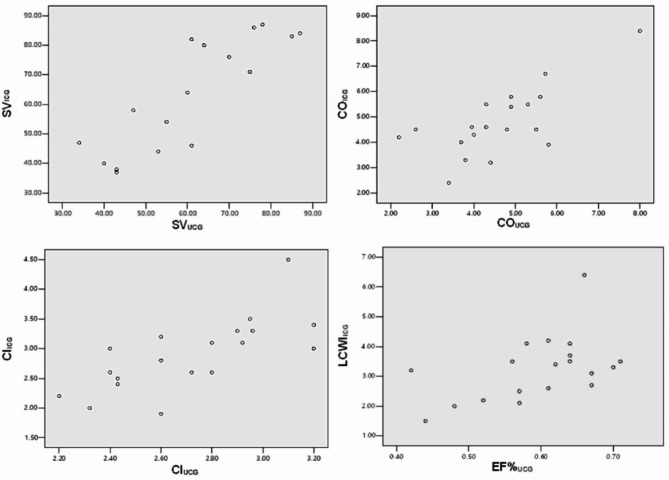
Correlation with SV, CO, CI and LCWI measured by ICG and UCG methods Abbreviations: *SV* Stroke volume, *CO* Cardiac output, *CI* Cardiac index, *LCWI* Left cardiac work index, *ICG* Impedance cardiography, *UCG* Ultrasound cardiography
